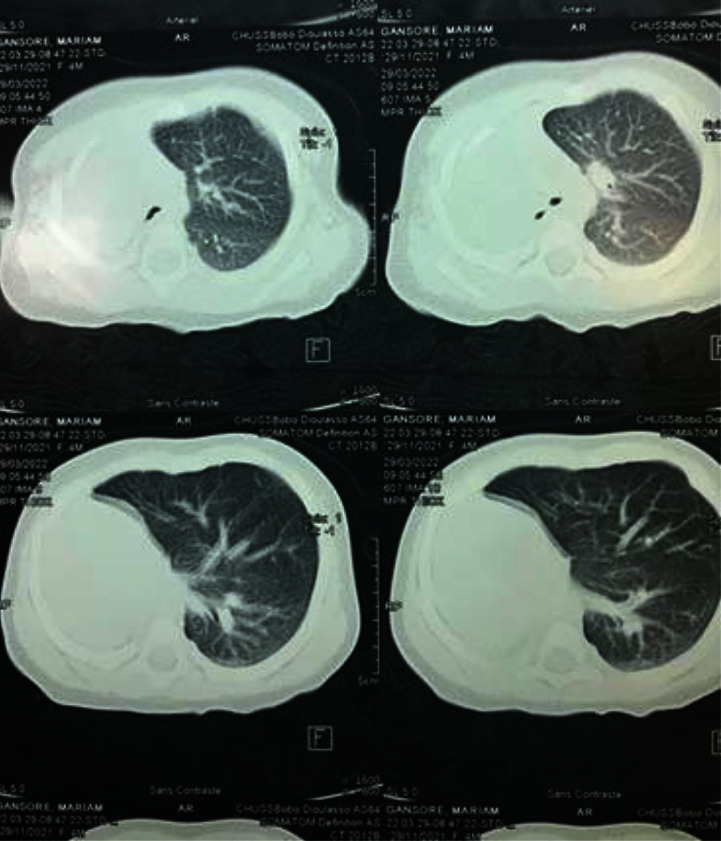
Chest CT scan in a 4-month-old infant showing agenesis of the right lung parenchyma, absence of a right stem bronchus, compensatory hypertrophy of the left lung, which extended to the right hemithorax, causing deviation of the mediastinum and responsible for dextrocardia, and a single left pulmonary artery.
